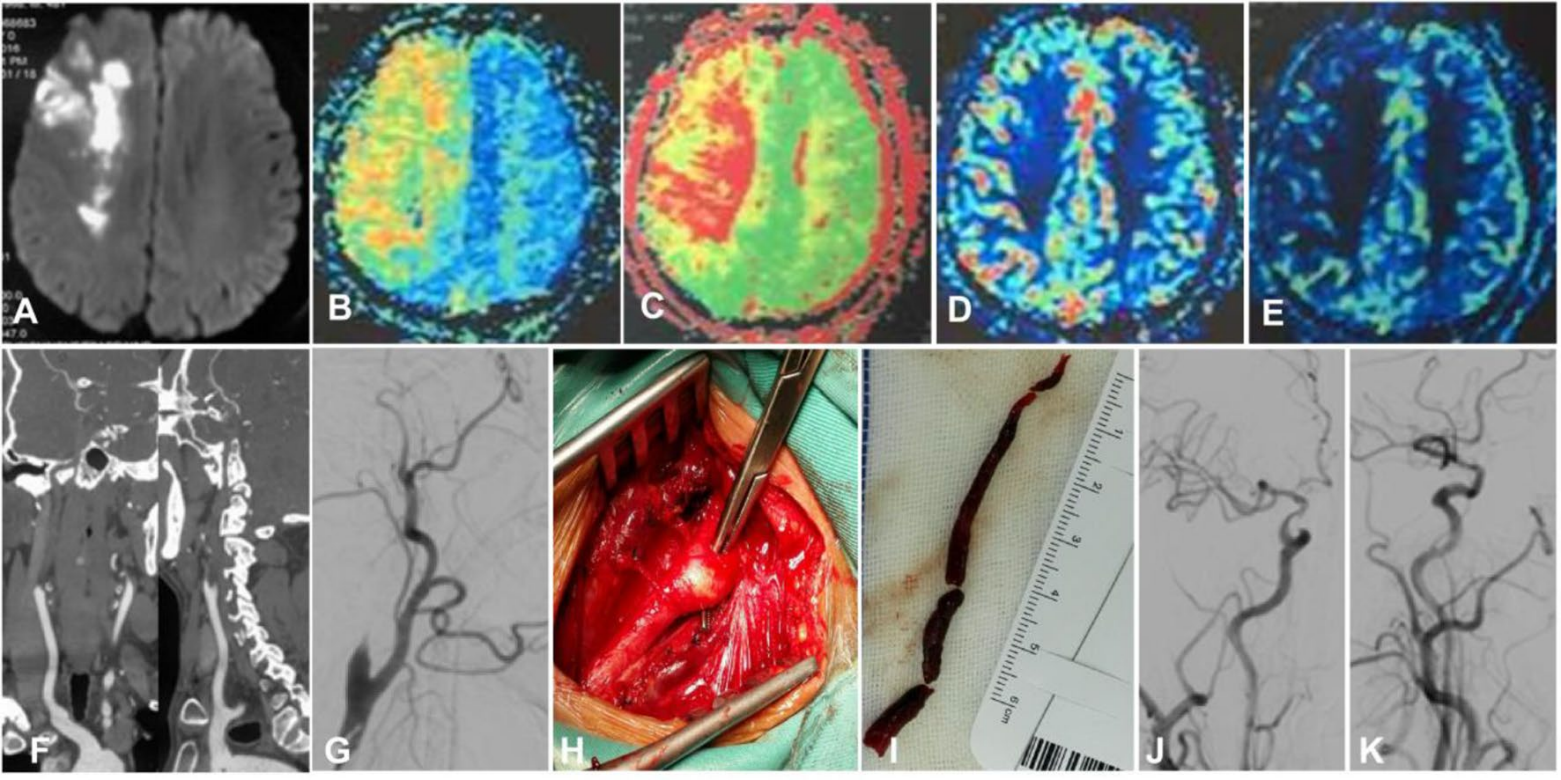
Distal atherosclerotic plaques causing long-segment occlusion of the internal carotid artery (ICA) treated with hybrid surgery in a patient in their 40 s with dizziness and left limb weakness. (A) Magnetic resonance imaging (MRI) showed multiple acute infarction lesions in the right hemisphere. (B–E) The time-to-peak (B) and mean transit time (C) were prolonged, and the cerebral blood volume (D) and cerebral blood flow (E) were decreased. (F) Computed tomography angiography revealed occlusion of the right ICA. (G) Digital subtraction angiography demonstrated occlusion of the right ICA. (H,I) Arteriotomy of the right ICA was performed for use of a Forgarty balloon to remove the atherosclerotic plaque (H), and the thrombus was approximately 7 cm long (I). (J,K) After plaque removal and recanalization of the ICA, a stenosis of the ICA ophthalmic segment was treated with balloon dilation, and smooth blood flow was resumed in the right ICA even though a slight stenosis remained at the ICA ophthalmic segment.
